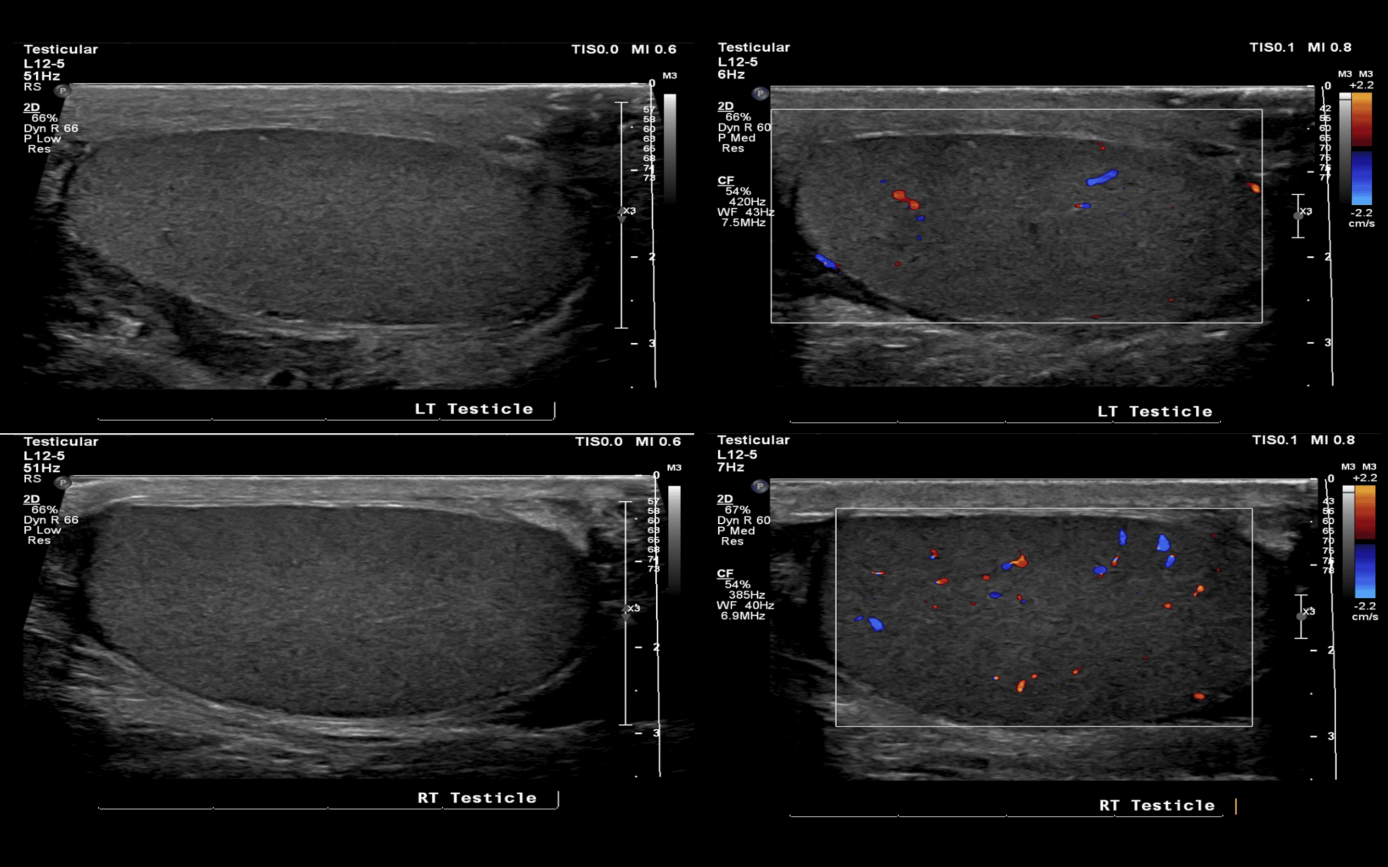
Follow-up ultrasound showing testicular vascularity to be preserved bilaterally
